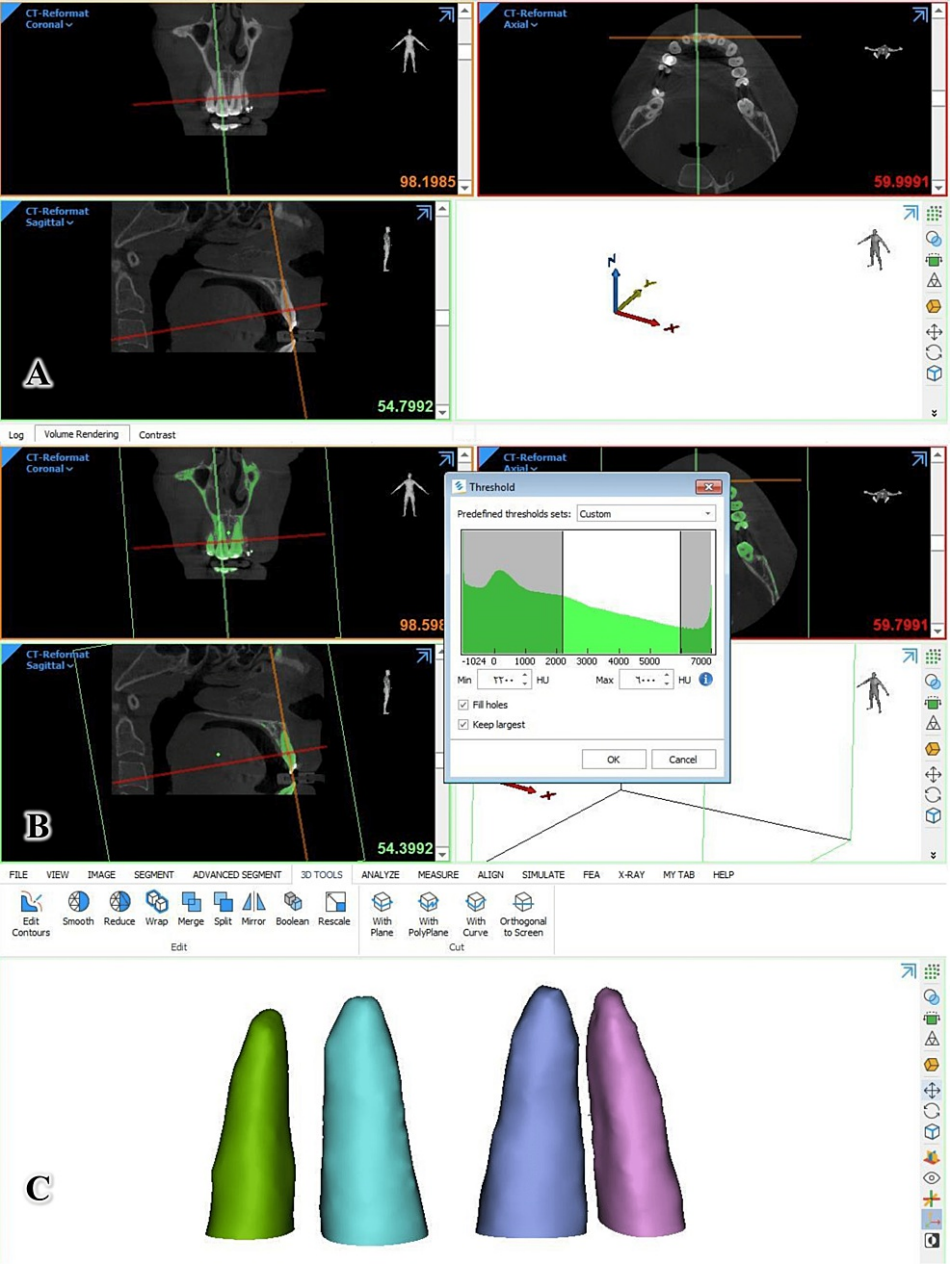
The software used to view and manipulate the DICOM files from CBCT Images. This software is also used to construct the roots of the upper incisor teeth. A: Orientation of the axes in the three dimensions. B: Determination of the appropriate intensity for each patient individually through the "Threshold" window. C: Once the 3D models of the upper incisors' roots were constructed, their volumes could be calculated DICOM: Digital Imaging and Communication in Medicine; CBCT: cone-beam computed tomography.
